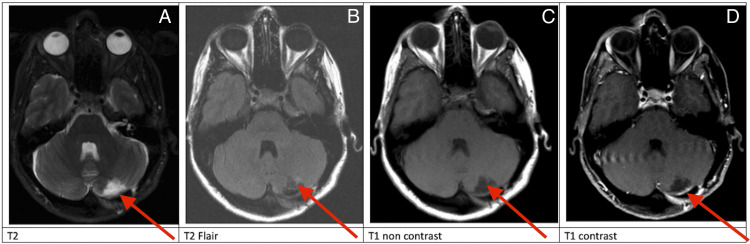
Three-month postop axial MRI Three-month postoperative MRI, red arrows (A-D) demonstrating no recurrent disease and stable resection cavity
